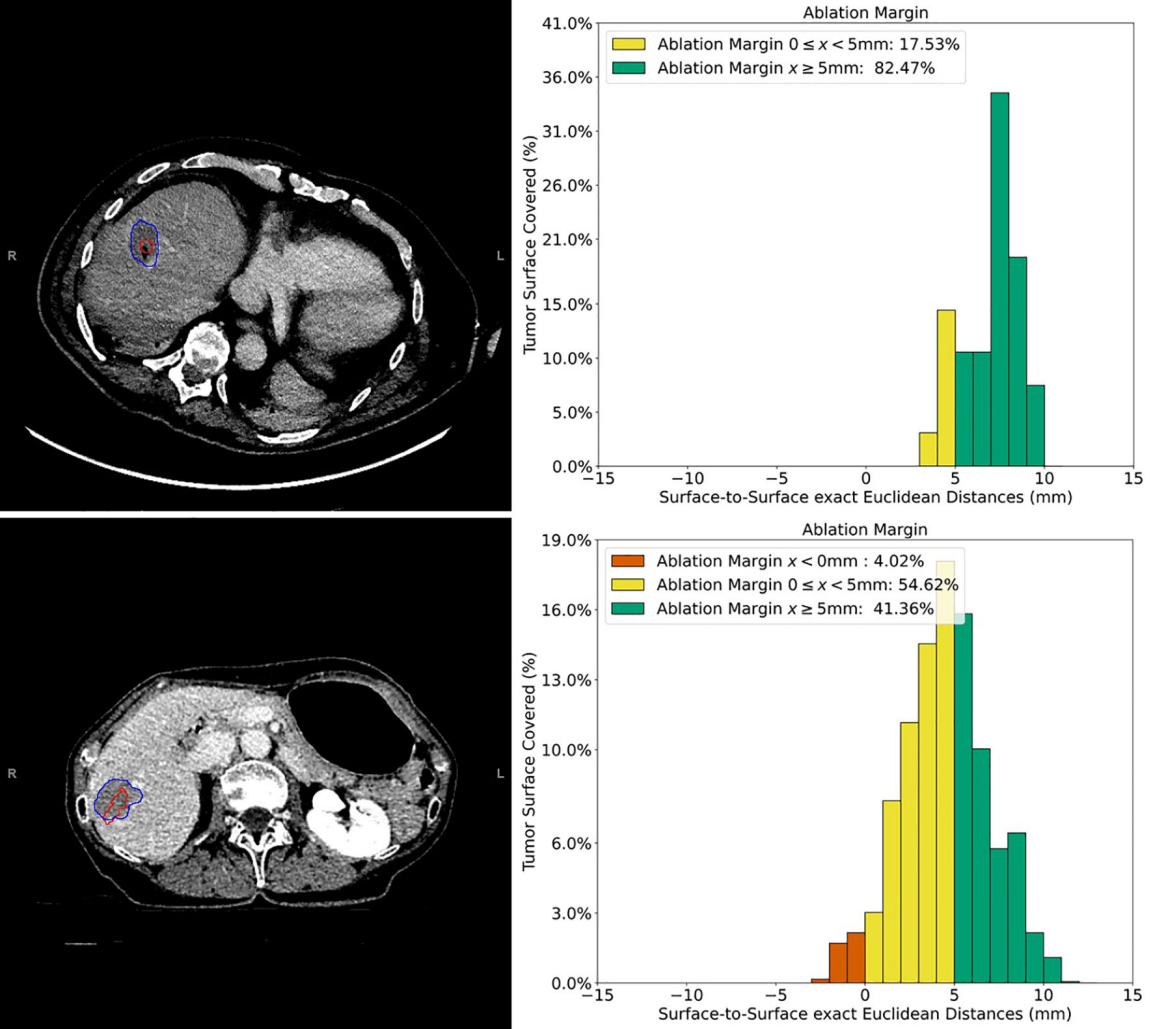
Example cases of three-dimensional quantitative ablation margin (3D-QAM) assessment in patients with colorectal liver metastases (CRLMs). Upper images: contrast-enhanced CT image of a CRLM in segment 8 treated with stereotactic microwave ablation (SMWA), with corresponding histogram of 3D-QAM on the right. Lower images: contrast-enhanced CT image of CRLM in segment 7 and its corresponding histogram of 3D-QAM distribution on the right. Segmentations of the tumor in both CT scans are marked in red, and the corresponding ablation volumes are marked in blue. Relative distribution of ablation margins with respect to percentage of tumor surface covered is displayed as a histogram, colored green for margins >5 mm, yellow for margins 0–5 mm, and red for residual tumor (margins <0 mm).
